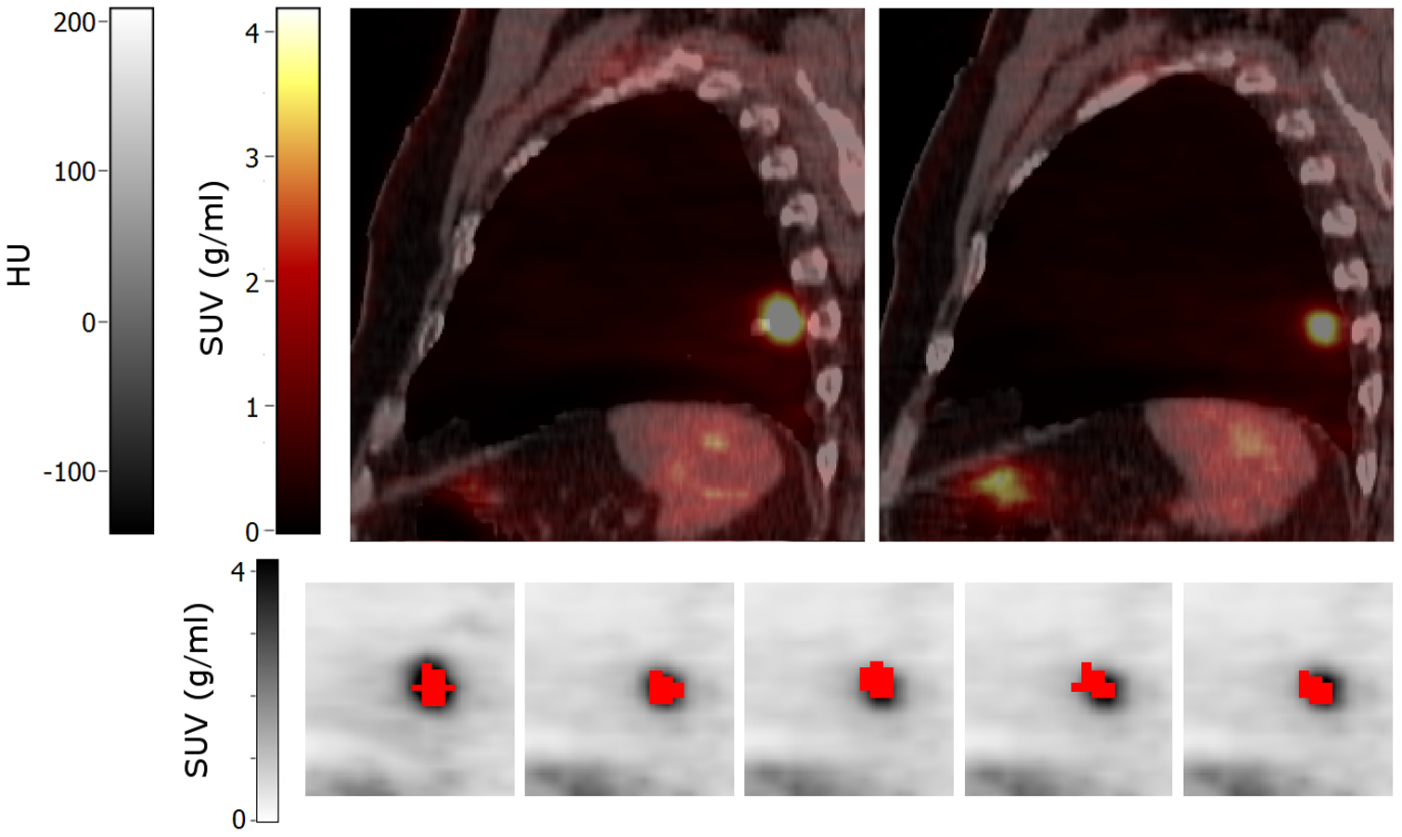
Sagittal images of a patient with a small lung metastasis. Top row: baseline (left) and early response (right) PET/CT images. Bottom row: volumes of interest (shown in red) projected onto the baseline (first image) and early response scans (other images) that were obtained using (from left to right): A50 defined on baseline scan, A50 defined on early response scan, and local rigid PET, non-rigid PET and non-rigid CTPET image registration. All images are shown using the same colour scales. Abbreviations: SUV, standardized uptake values; HU, hounsfield units; A50, 3 dimensional (semi-)automatic isocontour method at 50% of the maximum pixel value that corrects for local background.
